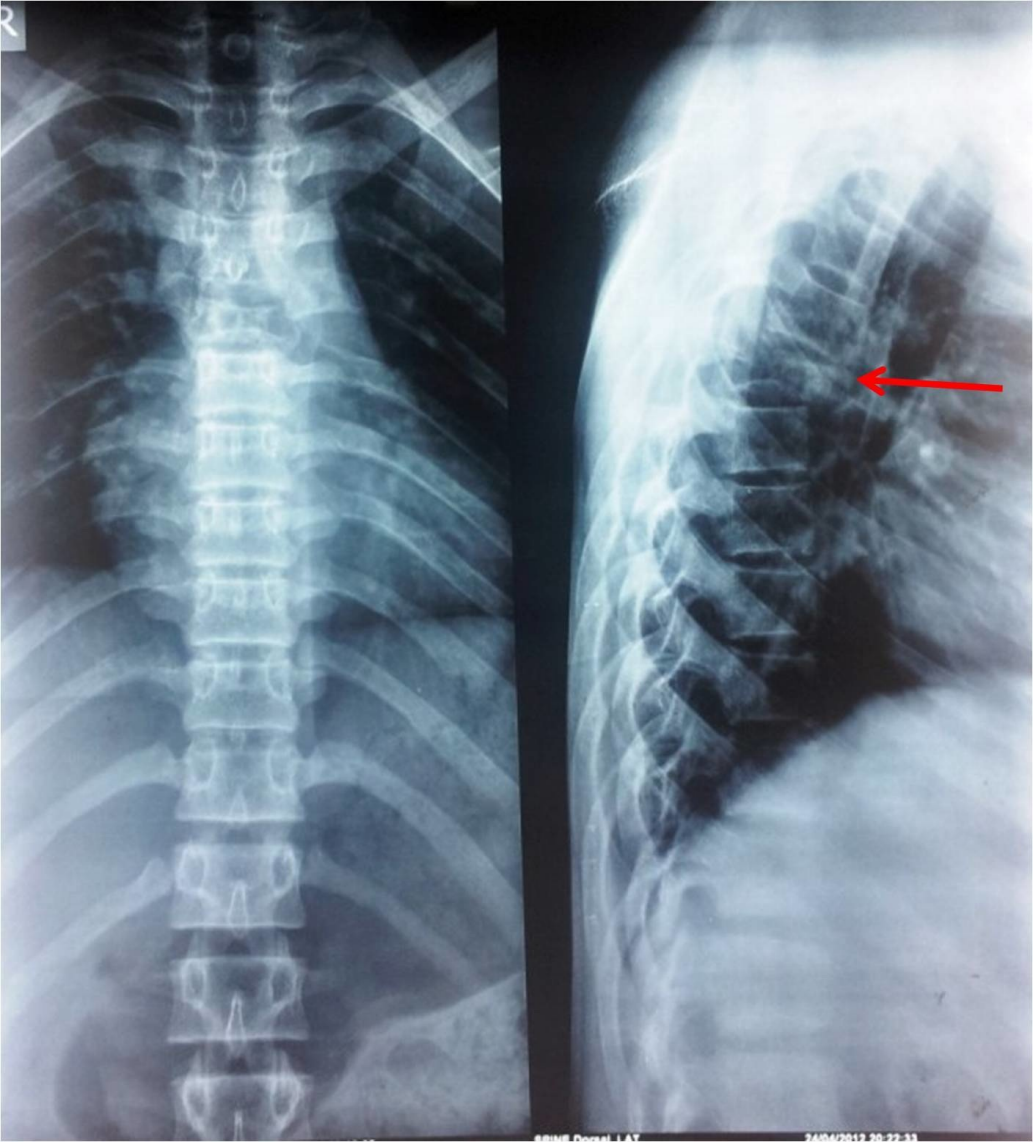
Radiograph showing collapse of T5 vertebra Anteroposterior and lateral view x-ray of the thoracic spine showing pathological fracture and collapse of T5.
